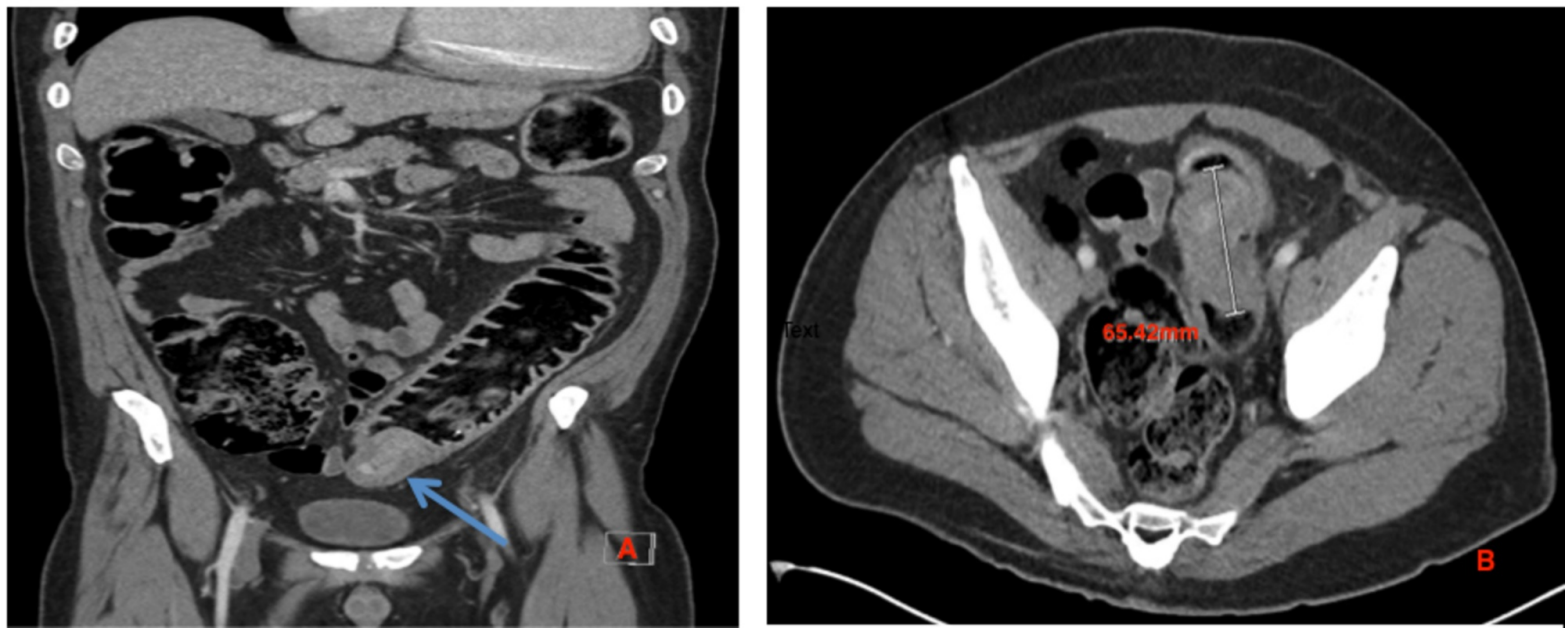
(A) A coronal view of CT of the abdomen and pelvis showing large obstructing mucocele (blue arrow). (B) An axial view of CT of the abdomen and pelvis showing a 65-mm mucocele.
